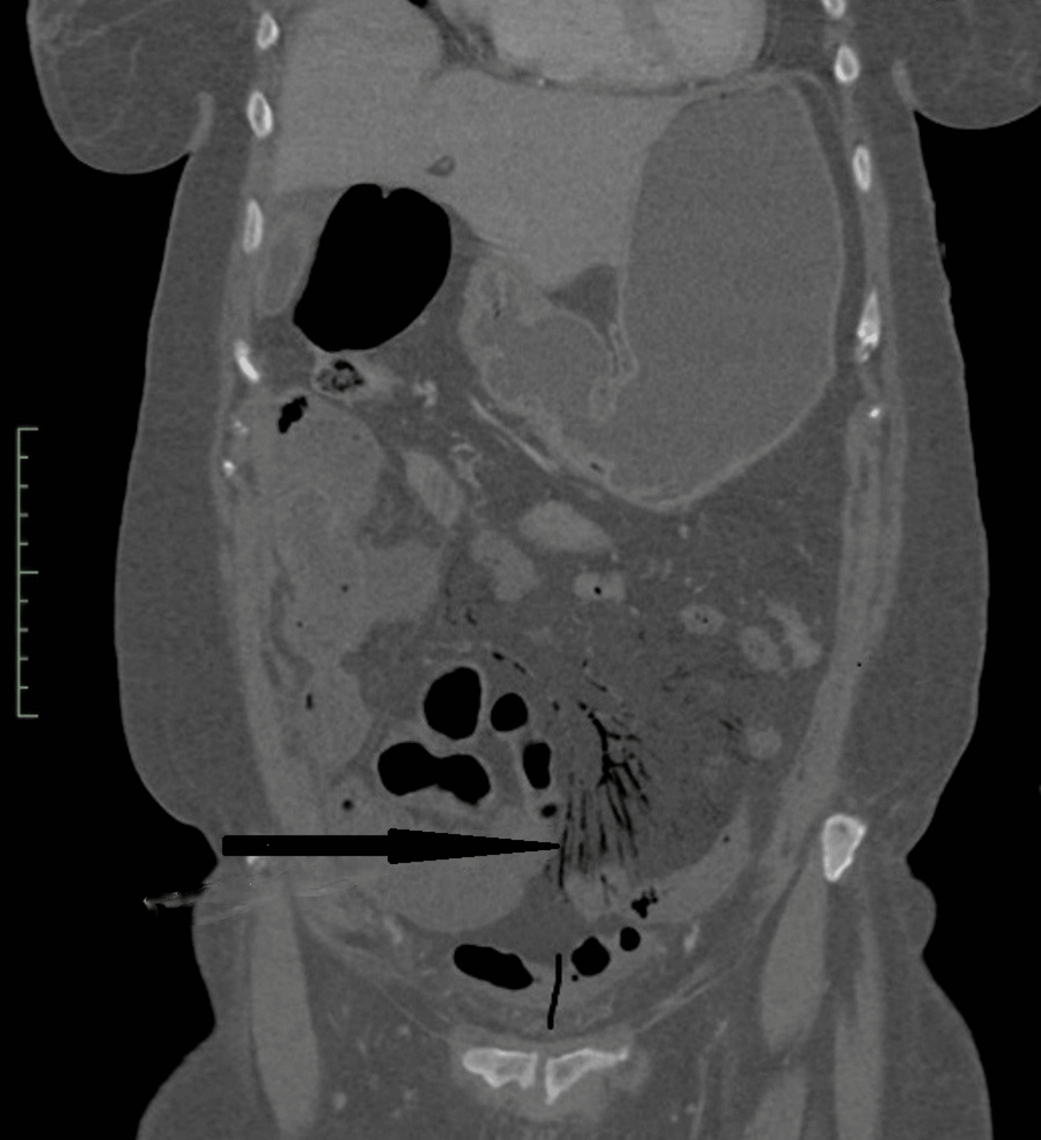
Coronal CT scan view of the abdomen and pelvis revealing mesenteric intravascular gas (black arrow)
